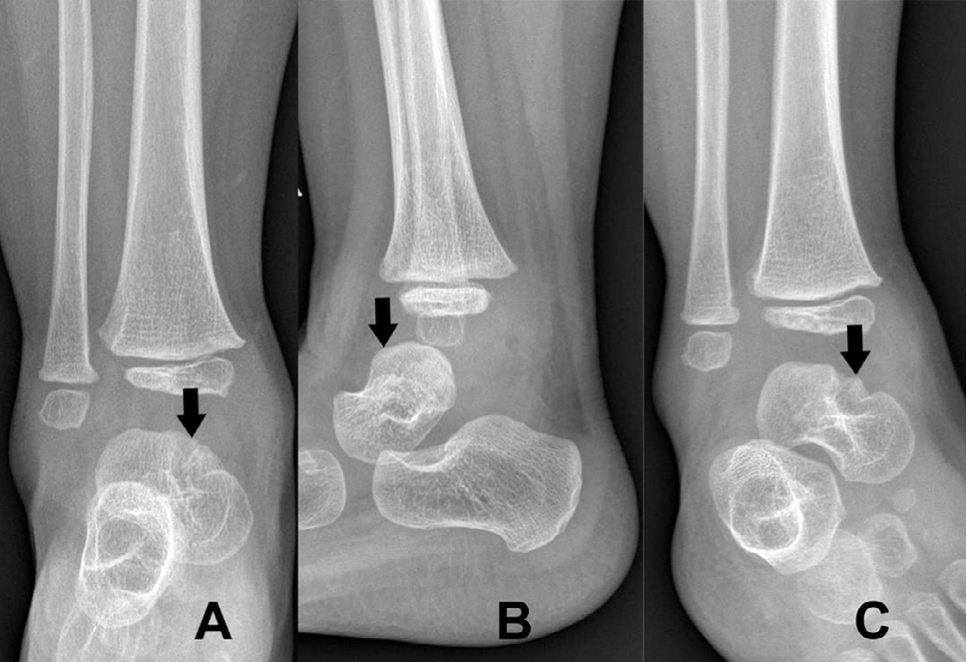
Radiographs of patient 1 at 12-month follow-up are demonstrated. The arrows represent an osteolytic lesion at the talus.
